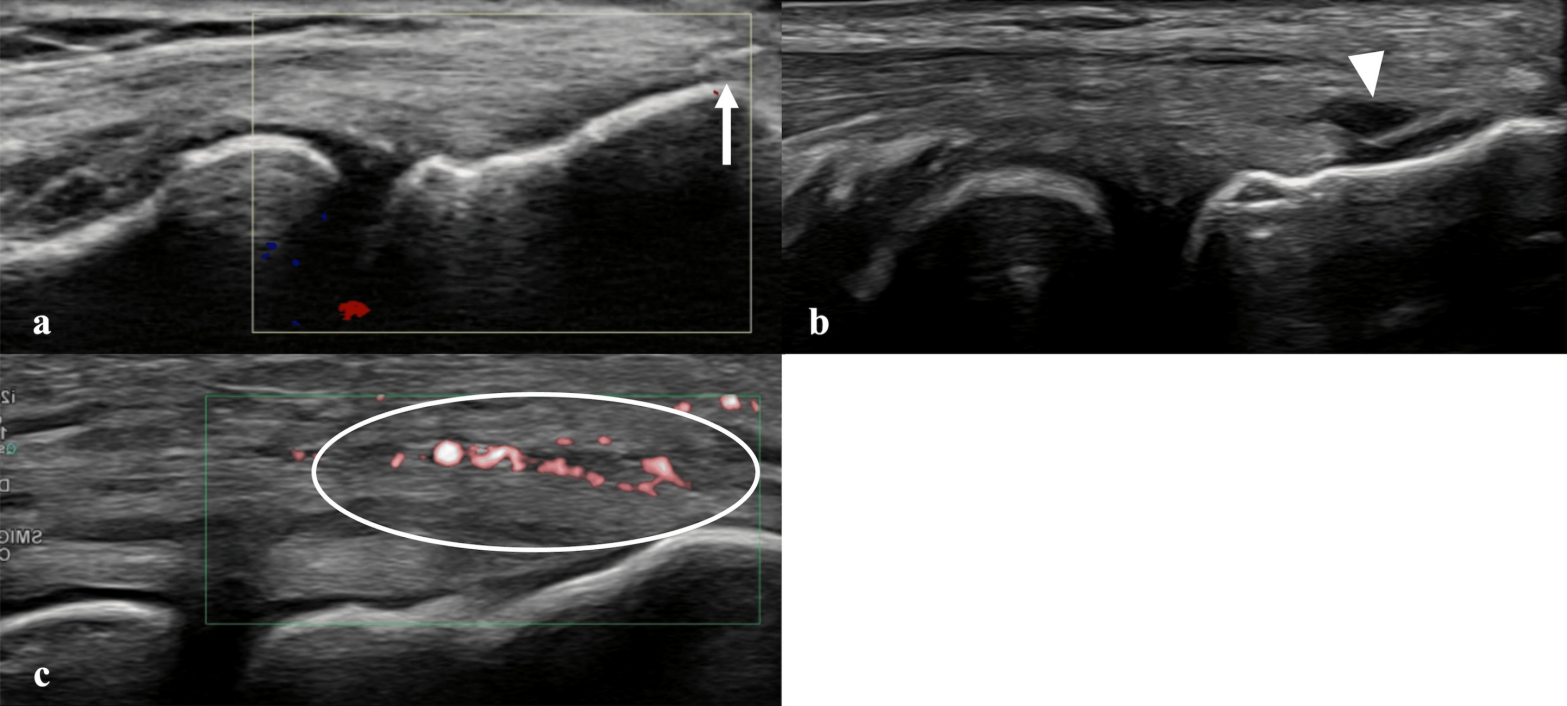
Ultrasonographic findings at the tendon insertion (a) Hyperechoic lesion at the lateral epicondyle suggestive of calcification (white arrow). (b) Hypoechoic lesion within the tendon suggestive of a partial tear (white arrowhead). (c) Tendon swelling and increased vascularity demonstrated by enhanced Doppler signal (white circle).
